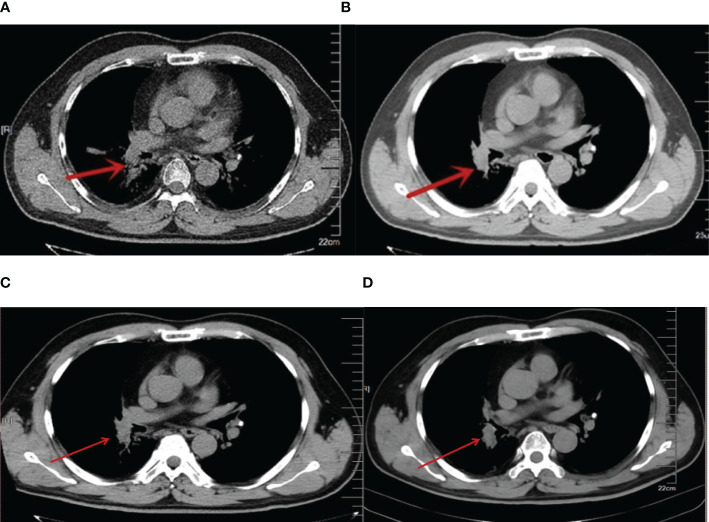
(A) 2013 lung CT, red arrow indicating more progressive lesions in the right lung (after chemotherapy). (B). 2013 lung CT, CIK cell therapy combined with chemotherapy after 3 months, the red arrow indicates a reduction in lesions in the right lung. (C). 2016 lung CT. CIK cell therapy combined with chemotherapy. Red arrow indicates lesions in the right lung. (D). 2023 lung CT. Red arrows indicate stable lesions in the right lung.
